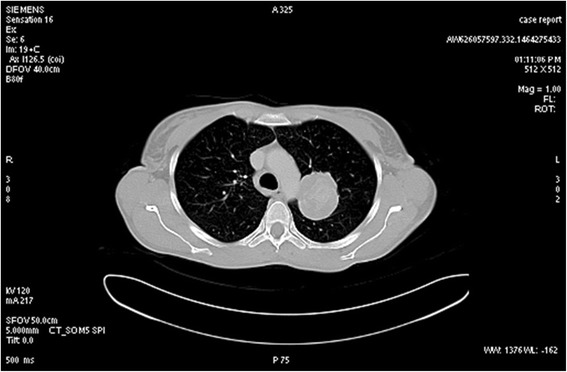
Thoracic CT scan showing a mass (50 mm of maximum diameter) in the upper left lobe
